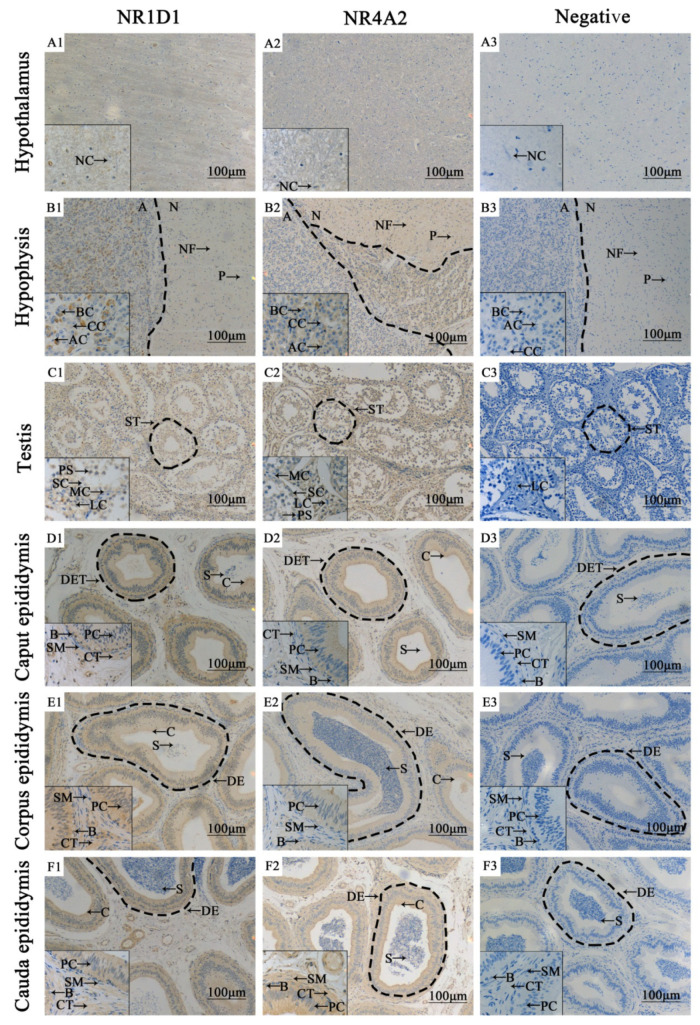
Localization of NR1D1 and NR4A2 proteins in adult male yak (4 years old) HPG tissues. (A1–F1), Localization of NR1D1 protein in adult male yak HPG tissues. (A2–F2), Localization of NR4A2 protein in adult male yak HPG tissues. (A3–F3), The negative control of adult male yak HPG tissues. NC, neurogliocyte (100×). The box in the lower left corner is a partial enlarged view (400×). A, adenohypophysis; N, neurohypophysis; P, pituicyte; AC, acidophilic cells; BC, basophilic cells; CC, chromophobe cells; NF, nerve fibers; ST, seminiferous tubule; MC, myoid cells; LC, interstitial cells; SC, spermatogonium; PS, primary spermatocyte; S, spermatozoon; DET, ductuli efferentes testis; B, basal cells; PC, principal cells; SM, smooth muscle cells; CT, connective tissue; DE, ductus epididymidis.
